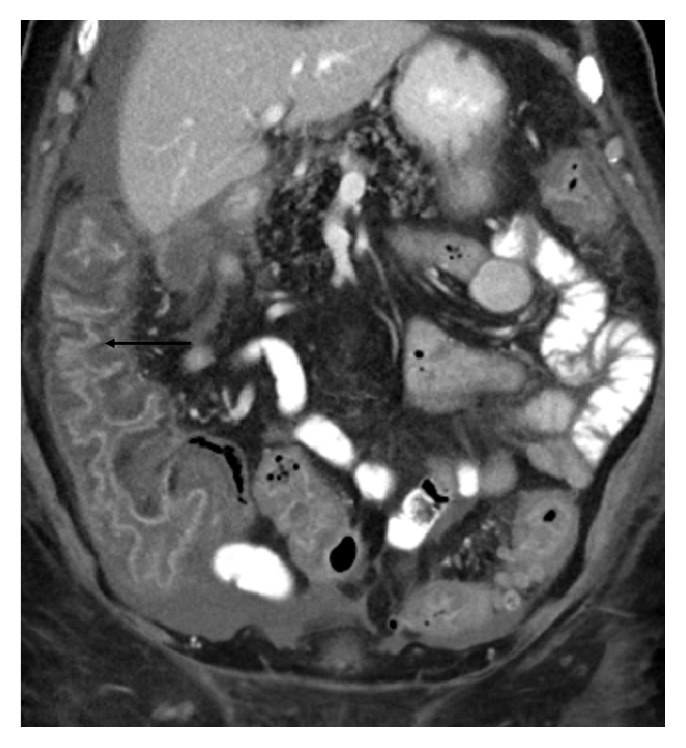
Sagittal view of CT scan of the abdomen showing ascending colon thickening (arrow).
